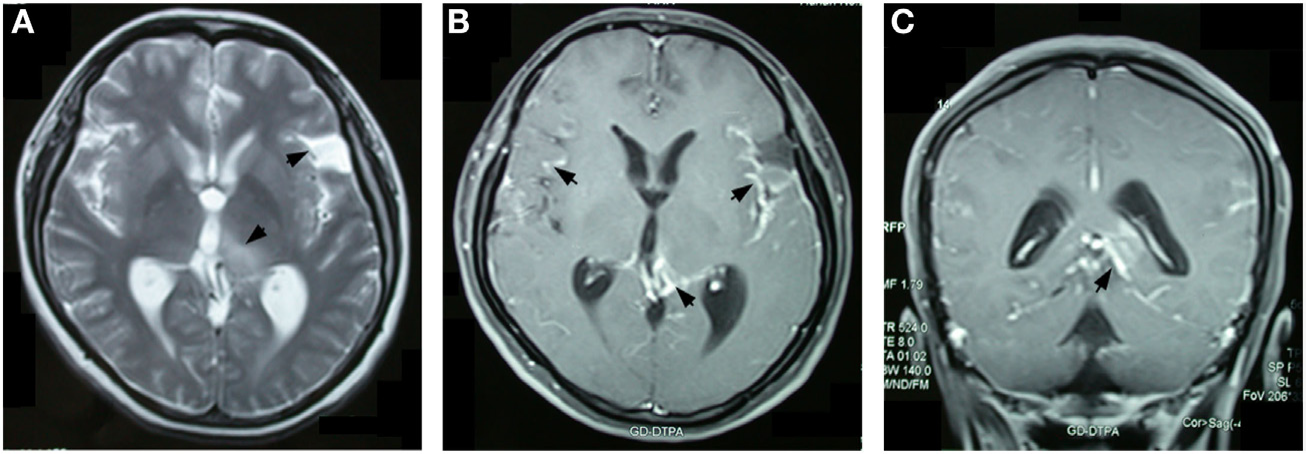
Brain magnetic resonance imaging 2 months after the onset of symptoms revealed an abnormal signal with hyper-density on T2-weighted images in the left thalamus and the dilated left Sylvian fissure (A). Overall enhancement was present in the cerebral meningeal, the cerebellar tentorium, the pia mater around left the thalamus, and the quadrigeminal cistern, but not in the left thalamus (B,C).
